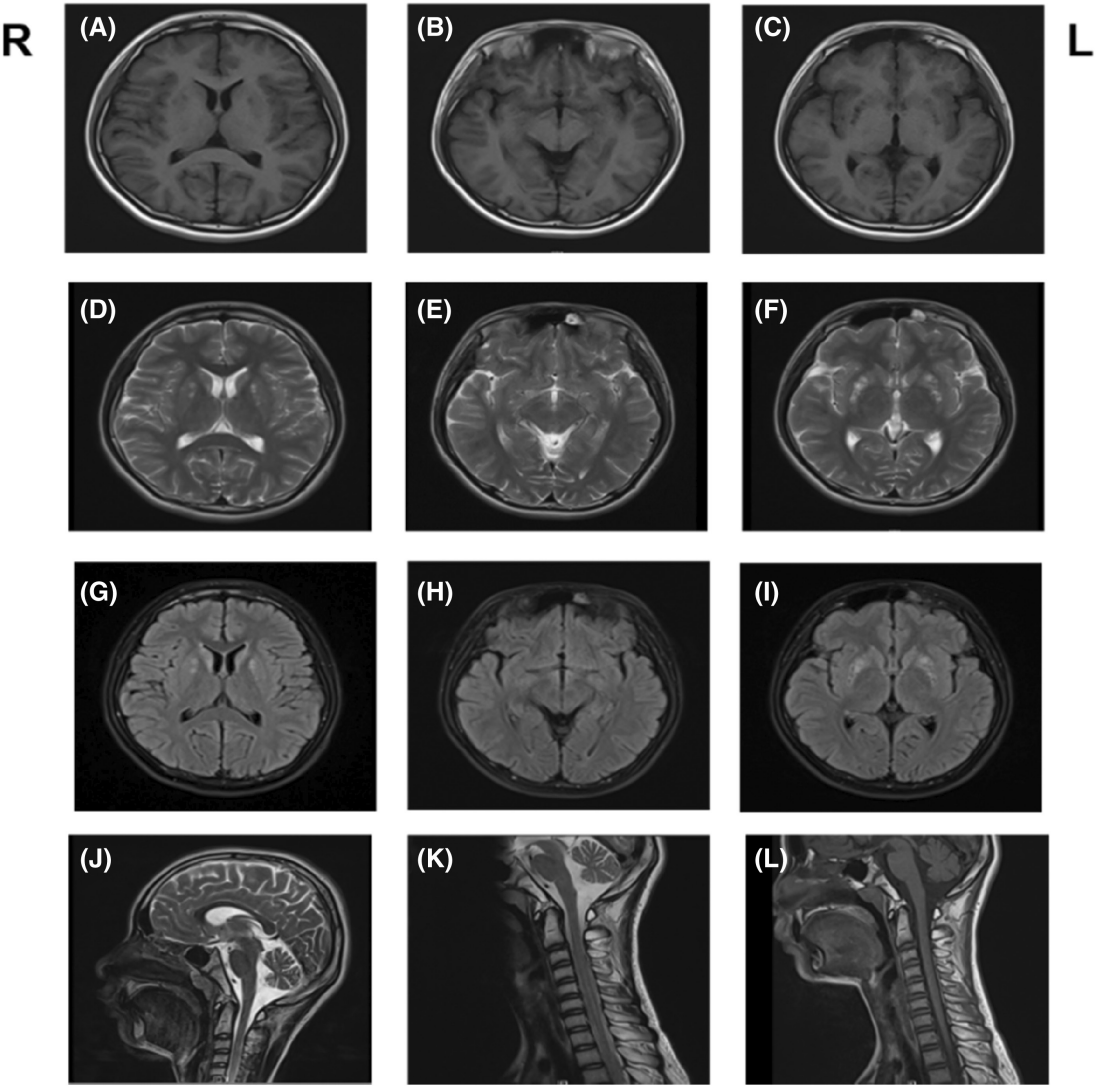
The patient's brain and cervical MRI. Axial T1‐weighted (A‐C), T2‐weighted (D‐F), and Flair (G‐I) MRI shows multiple abnormal signal changes in bilateral basal ganglia. Sagittal T2‐weighted (J) MRI shows a higher signal in the medulla. Sagittal T2‐weighted (K) and T1‐weighted (L) MRI shows abnormal signal changes in cervical cord 2–7.
